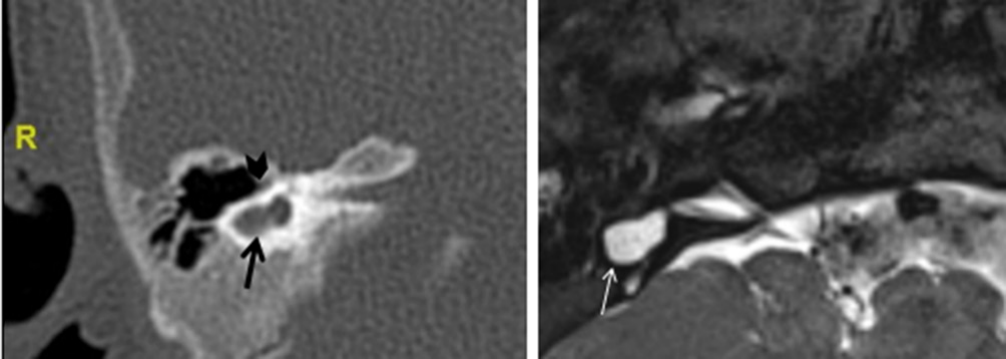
Cochlear aplasia on CT (left) and MRI (right) on axial planes. Note dysmorphic vestibule (arrows) and absent cochlear promontory (arrowhead) in keeping with cochlear aplasia with a dilated vestibule.
